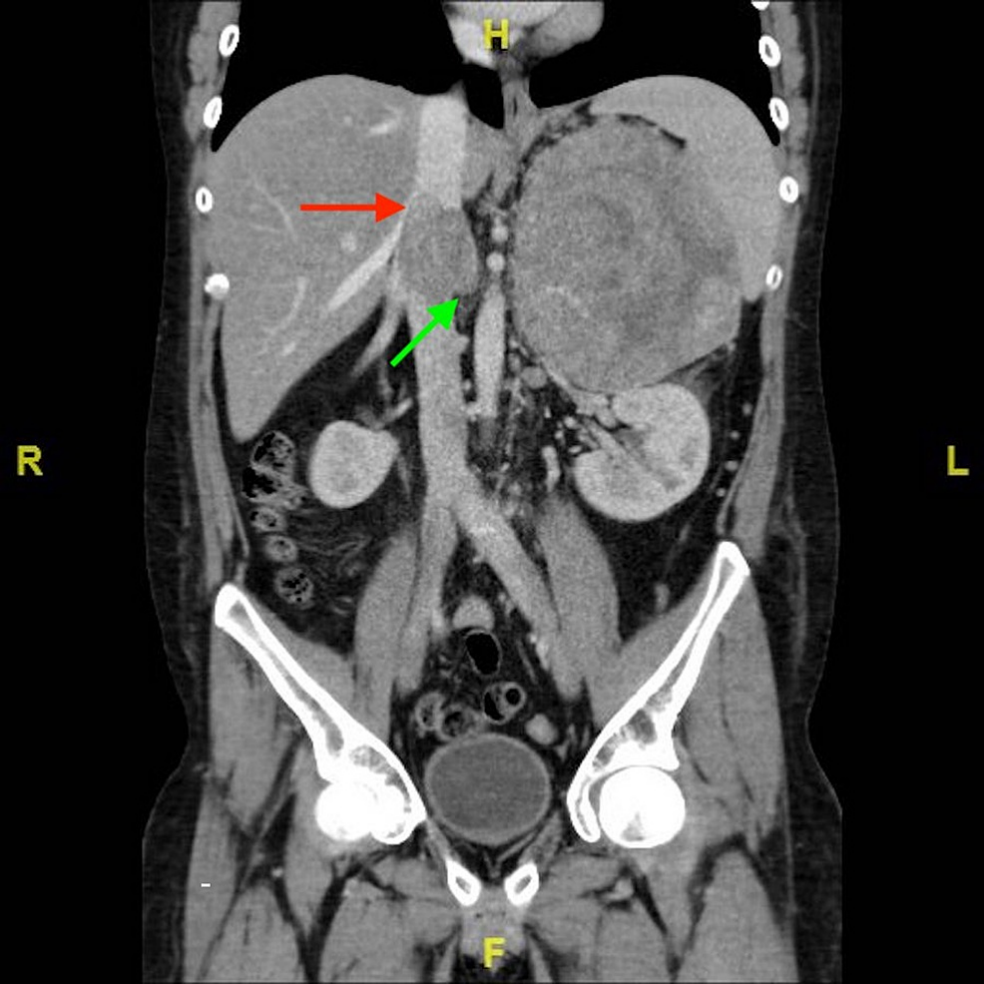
CT scan showing tumor thrombus extending into IVC (red arrow) and left renal vein (green arrow). IVC: Inferior vena cava.
